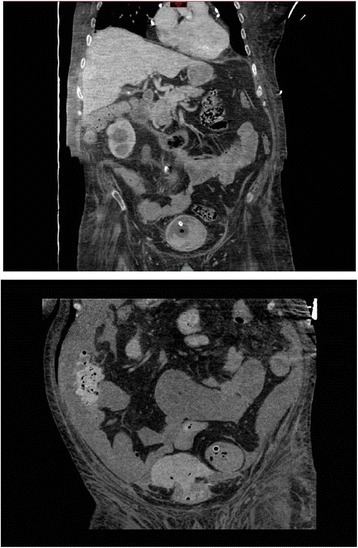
Top panel: Coronal section of CT of abdomen performed five days after vesical lithotripsy showed urinary bladder almost filled with clots. The outline of urinary bladder was intact. Bottom panel: Sagittal section of CT abdomen with oral positive contrast, performed 12 days after vesical lithotripsy (two days after cystoscopy and clot evacuation) when the patient developed abdominal distension: A large intra-peritoneal haematoma, at the superior aspect of dome of urinary bladder was seen with gas locules. There was a defect in the urinary bladder wall superiorly, at the site of the haematoma, indicative of bladder rupture/perforation.
